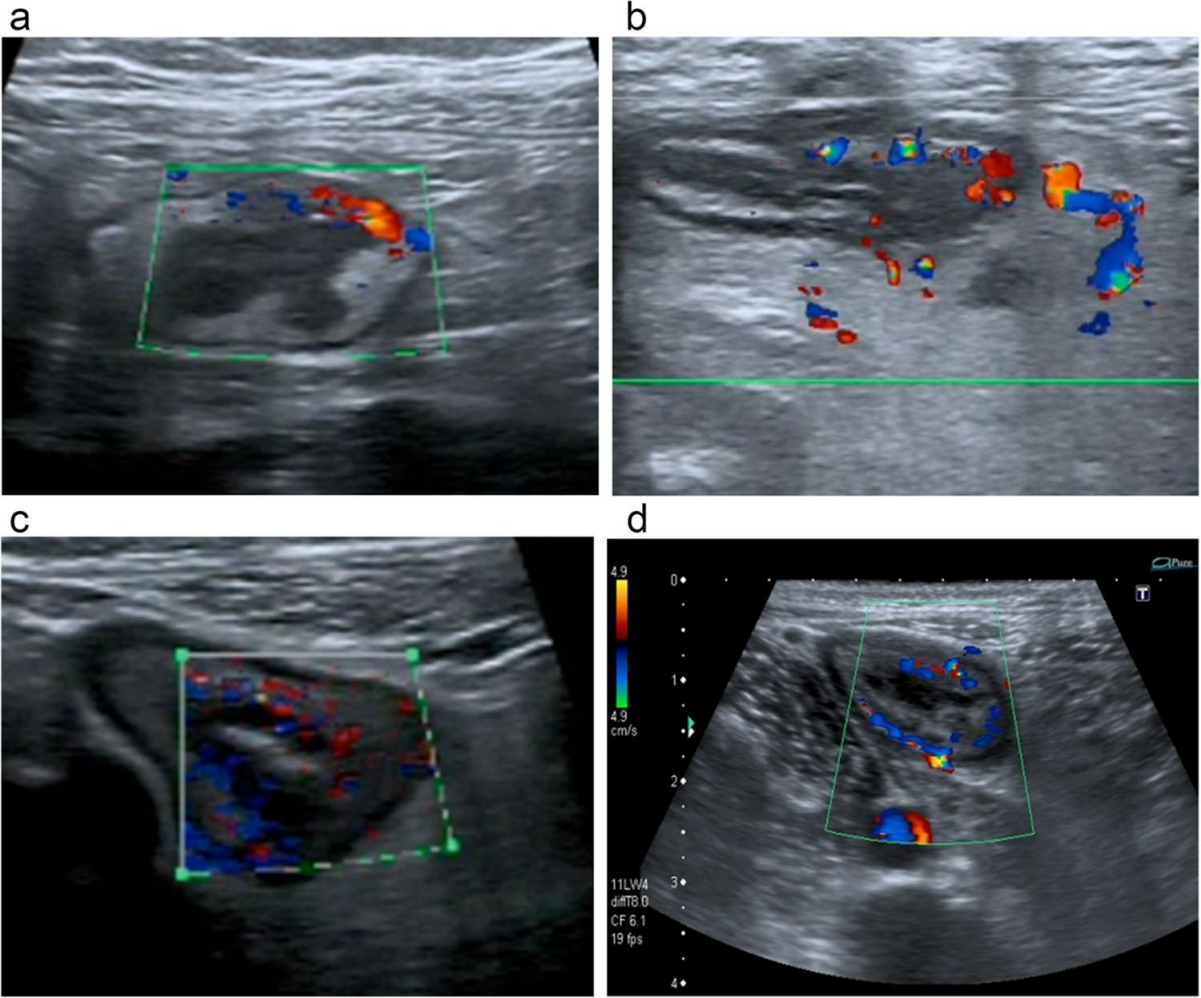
a, b Increased focal Doppler signal isolated to less than half the circumference on a trans-axial image compared to normal bowel in the same patient. c, d Increased generalised Doppler signal affecting more than half the circumference on a trans-axial image compared to normal bowel in the same patient
